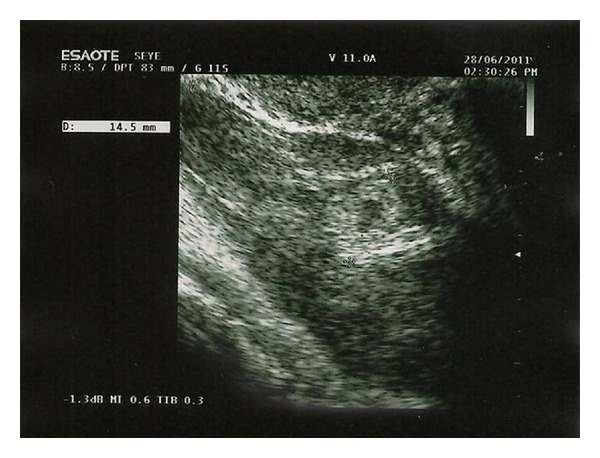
Transvaginal ultrasound of uterus showing left ovaric mass containing ovular material.
